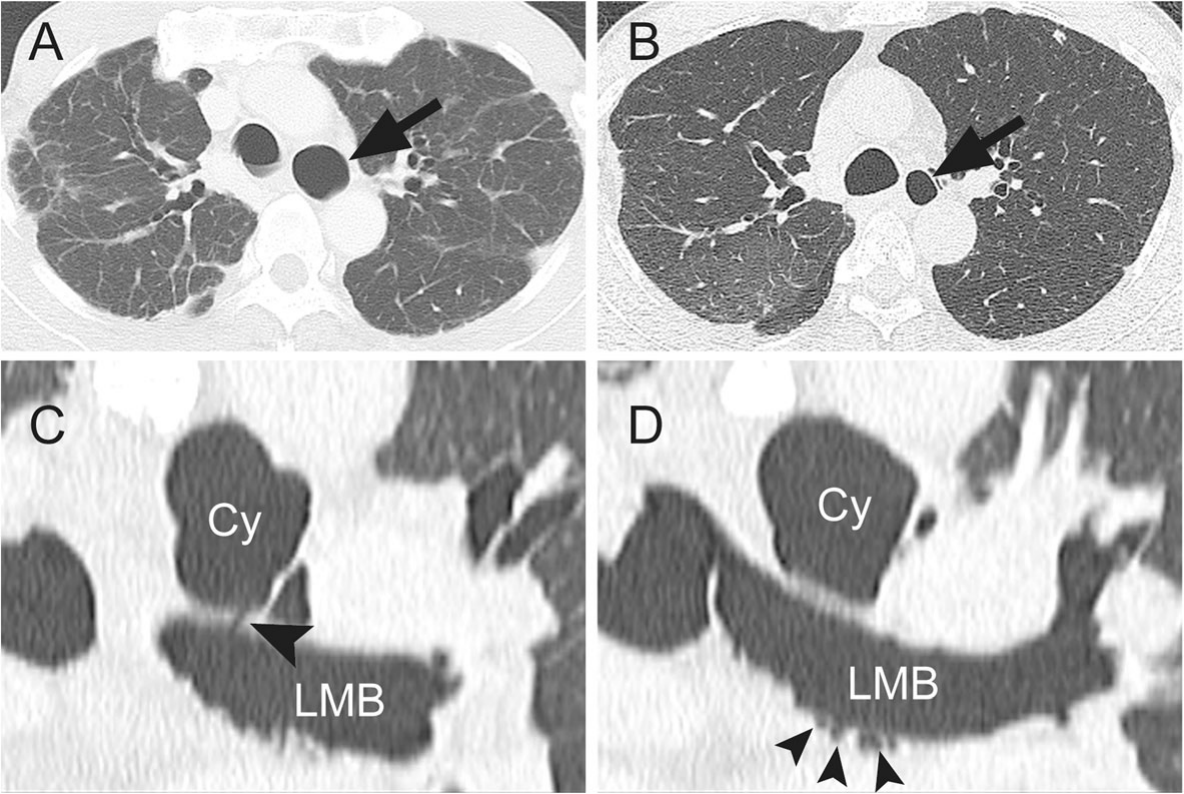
a Computed tomography (CT) showing a mediastinal air cyst (*arrow*) and fibrotic changes in the subpleural region. b CT obtained 4 years before the first visit to our hospital showing a smaller air cyst (*arrow*). c CT in the frontal plane showing a connection between the cyst (Cy) and the left main bronchus (LMB) (*arrowhead*). d CT in the frontal plane showing multiple extensions from the LMB (*arrowheads*)
